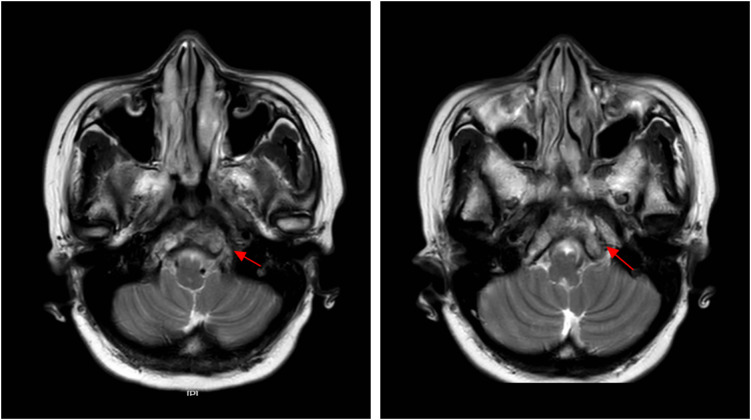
One month post-radiotherapy follow-up head MRI (T2W-mDIXON TSE sequence): Compared with pre-treatment images, the clivus lesion shows a reduction in size and a decrease in signal intensity on the T2W-mDIXON TSE fat-suppressed sequence (red arrows). No evidence of disease progression is observed.
